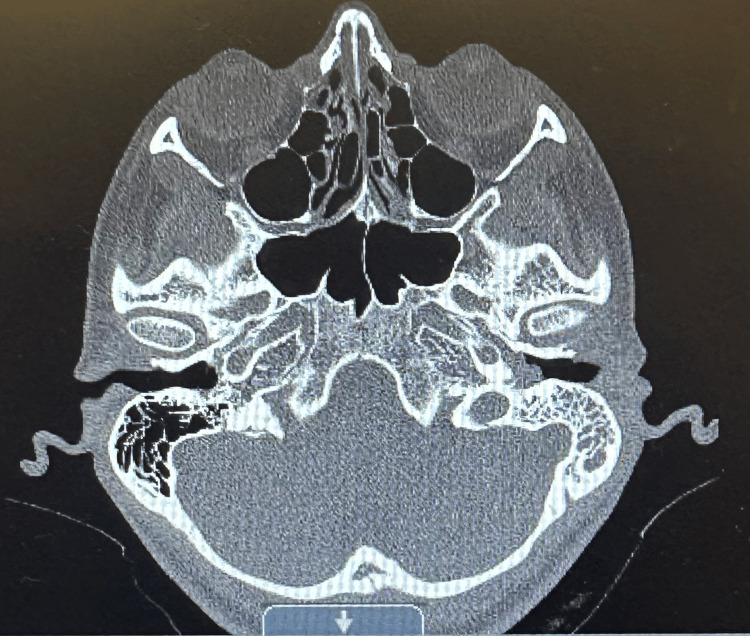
CT of the orbit/stella/posterior fossa without contrast
